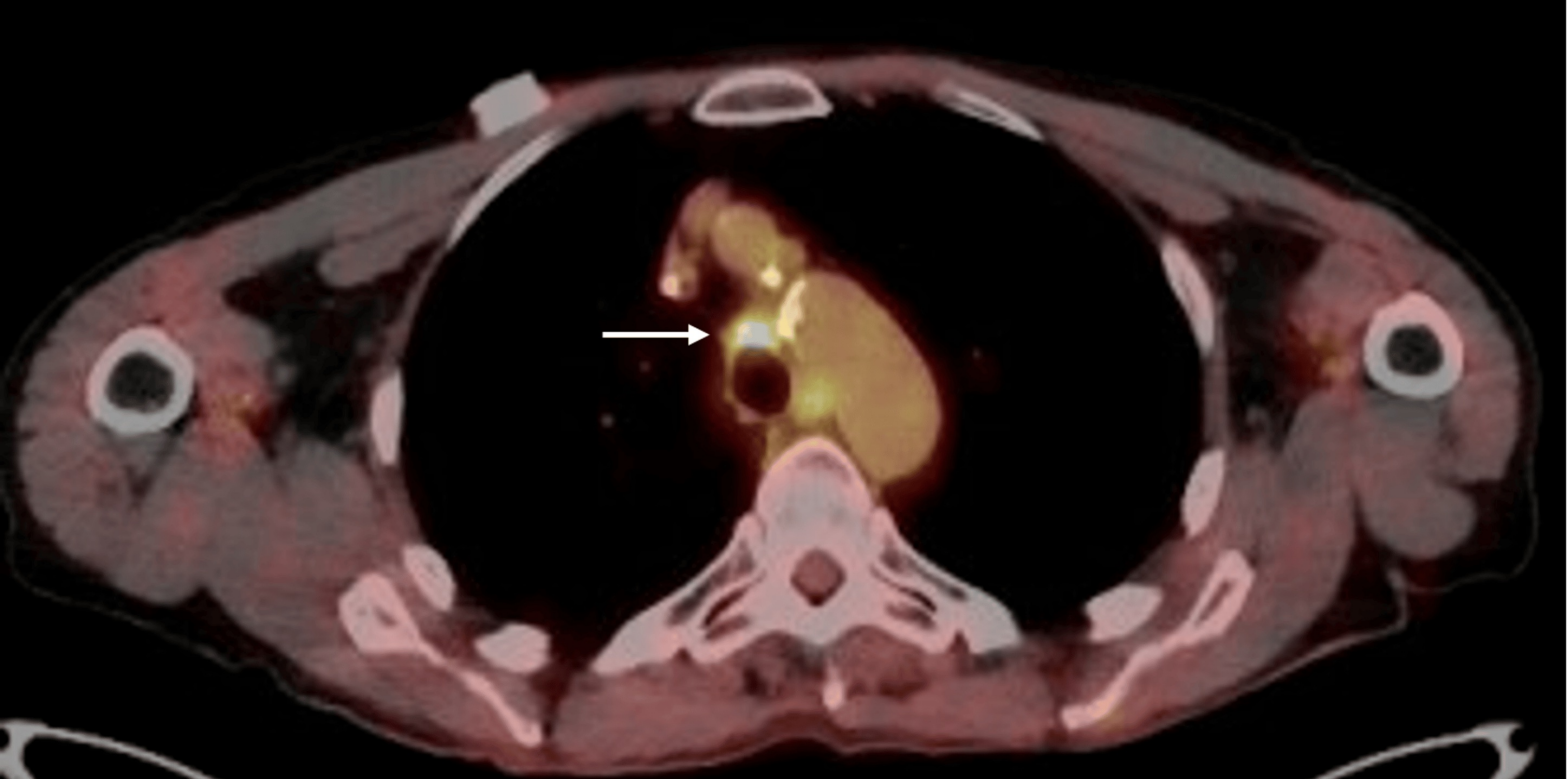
Axial PET/CT of the chest with FDG-18 3 months post-chemoradiation shows a decrease in size of the hypermetabolic mass with an SUVmax of 4.5
